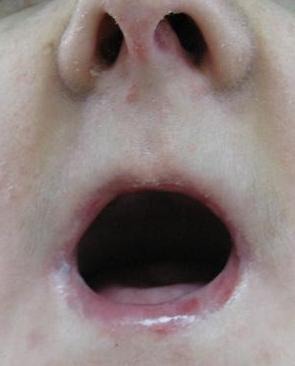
Two treatment options were considered: complete dentures and implant supported overdentures; treatment with dental implants was excluded because of volumetric deficiencies of bone in the CT scan analysis (Figure 2). Therefore, the selected treatment was conventional complete denture for upper and lower jaws.
